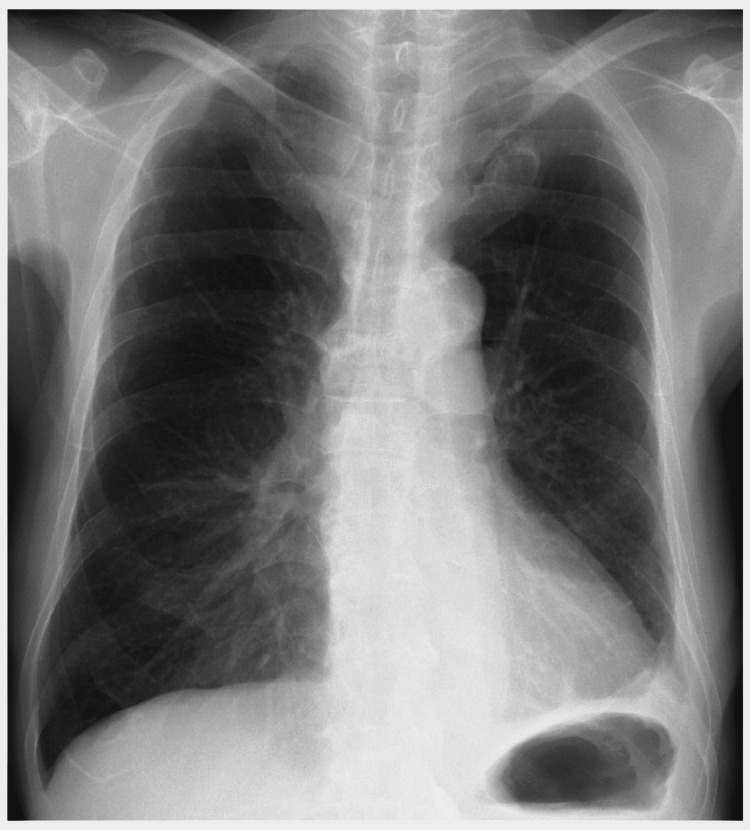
Chest radiograph finding after surgery The chest tube intruding into the left lung parenchyma was successfully removed, and pyothoracic cavities were decorticated.
